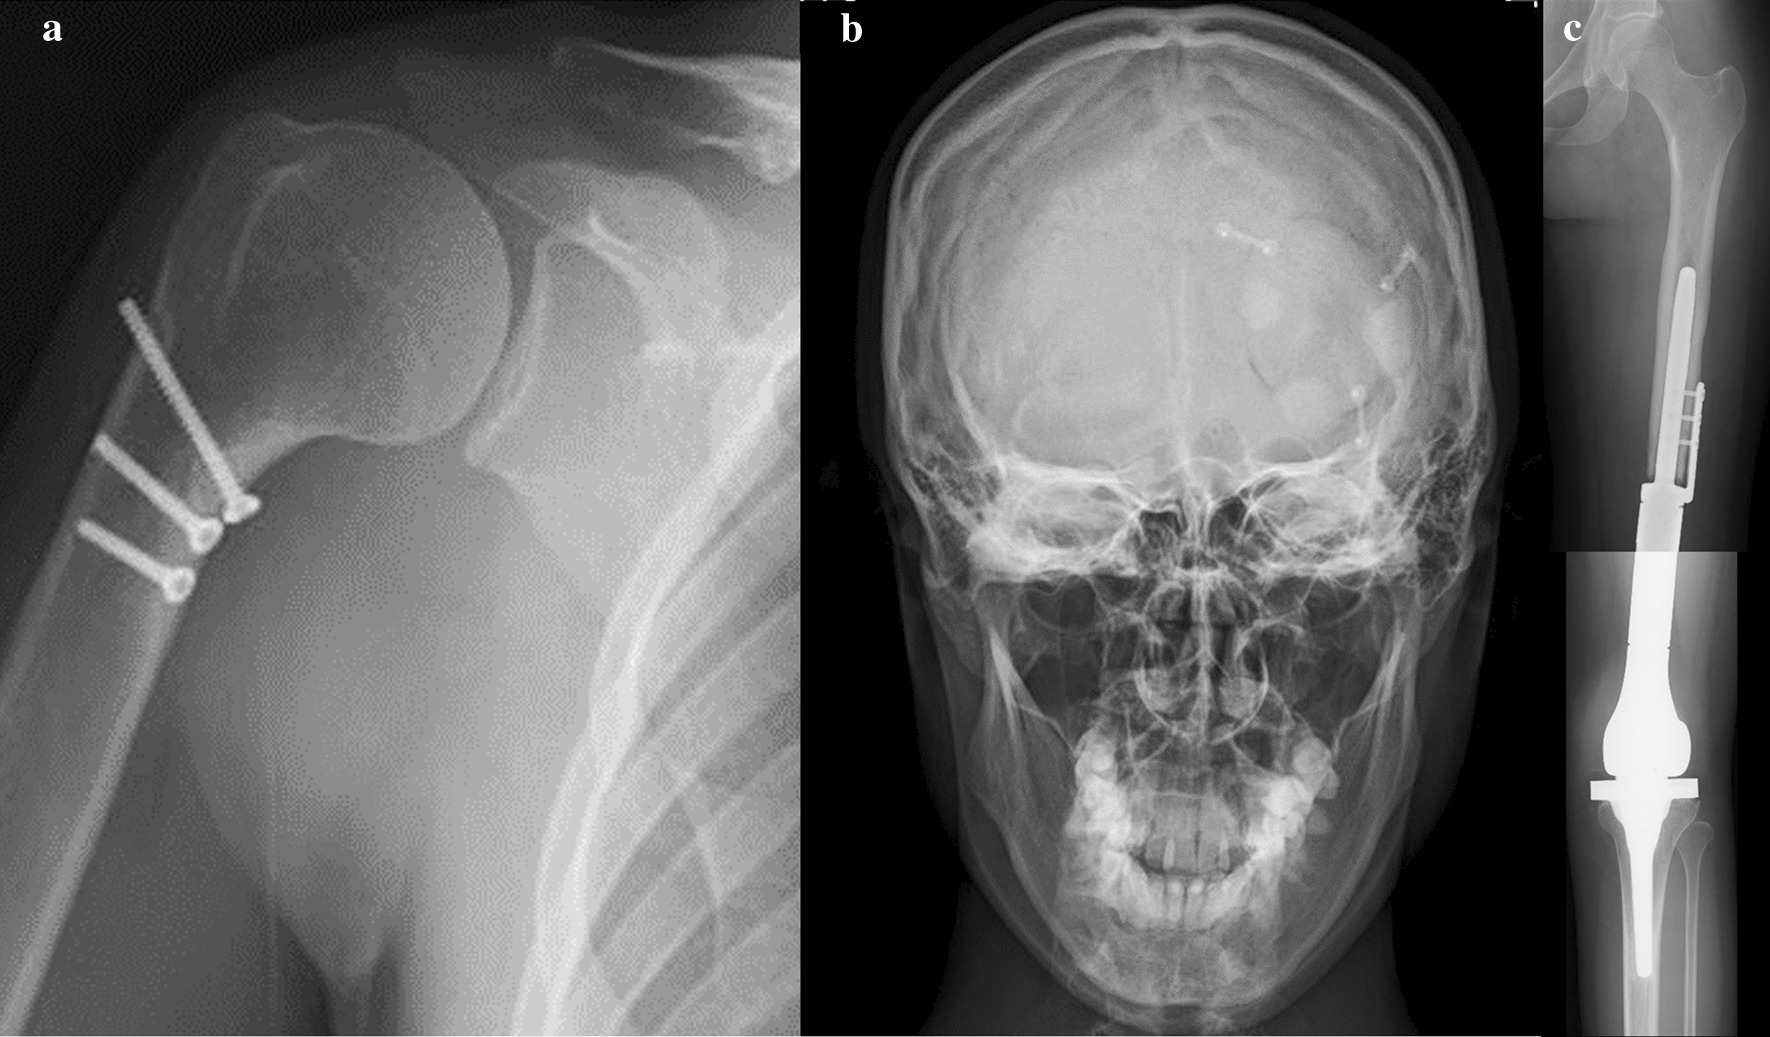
The postoperative roentgenogram at the latest follow-up. a Bone union at the osteotomy site for proximal humeral lesion, was completely achieved. b Bone union at the osteotomy site for skull lesion, was achieved. c Postoperative complications were not observed after tumor prosthesis reconstruction of the left distal femur
